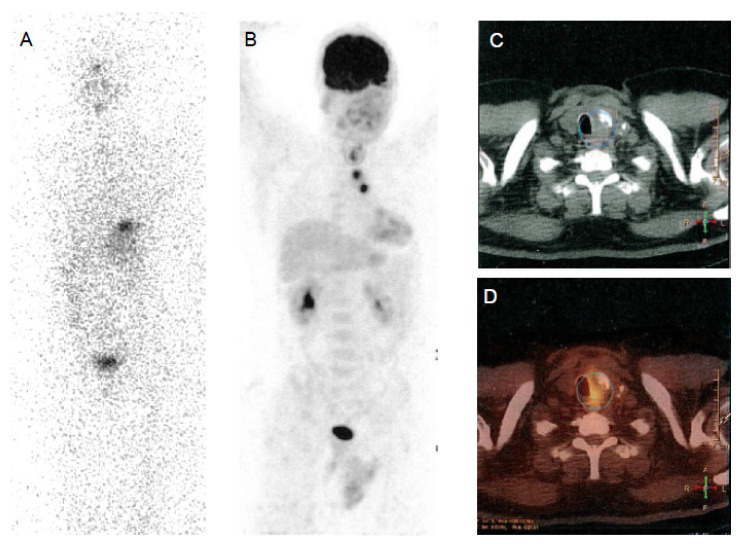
Papillary thyroid cancer in a 30-year-old woman. Post-therapy (A) ^131^I whole-body scan and ^18^F-FDG PET-CT (B,C,D) images. Post-therapy ^131^I whole-body scan did not show areas of uptake (A). ^18^F-FDG PET-CT images evidenced multiple areas of increased uptake in the left retrosternal and latero-cervical region.
